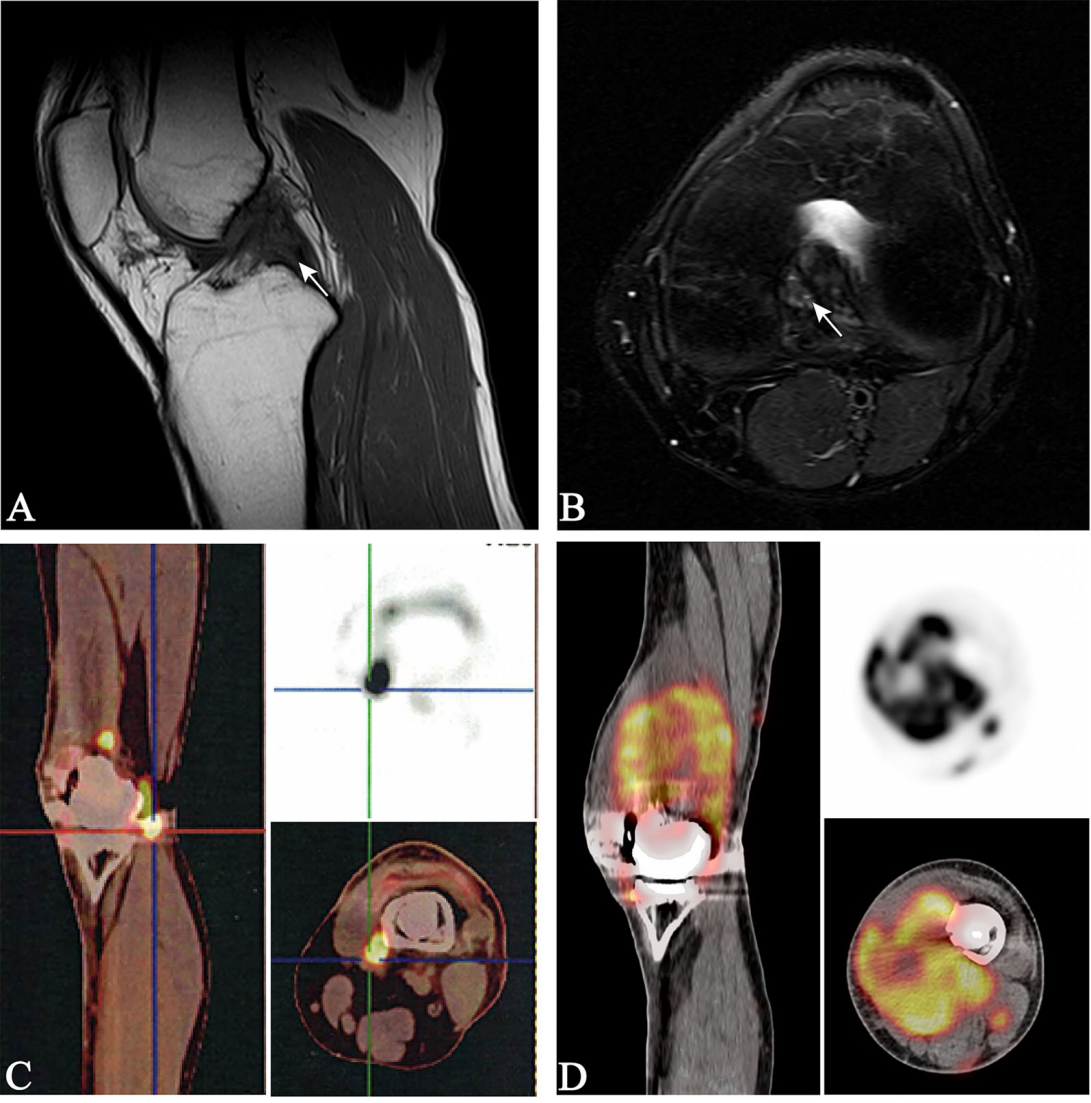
Magnetic resonance imaging of the left knee (A, B), white arrows indicate a mass measuring 2.8 × 3.8 cm violating the cruciate ligaments; positron emission tomography-computed tomography (PET-CT) (C), the fluorodeoxyglucose-avid tumour is seen within the left knee, indicating tumour recurrence; PET-CT (D), the fluorodeoxyglucose-avid tumour is presented on maximum intensity projection image as increased uptake around the left knee, indicating a second tumour recurrence.
